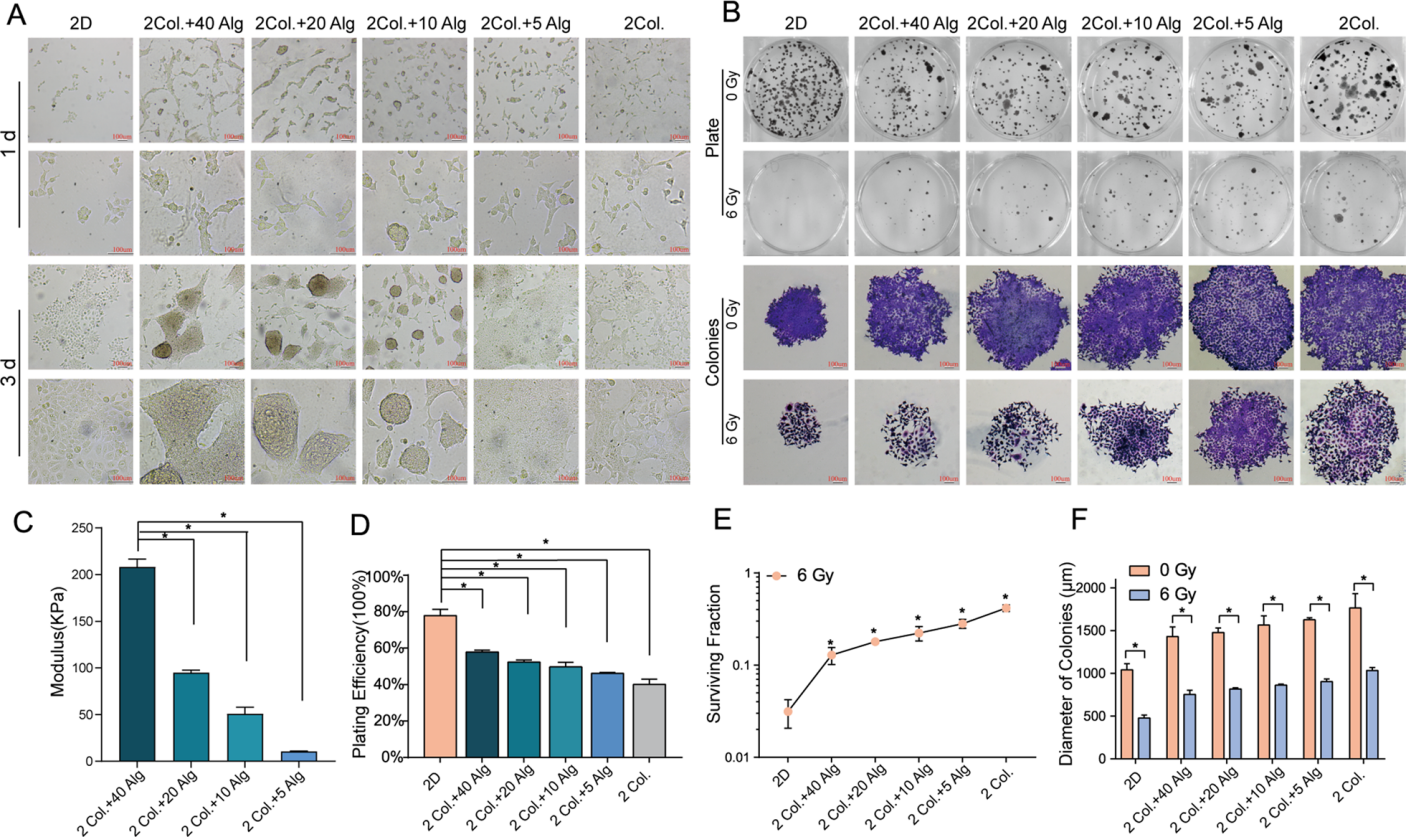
Radiation resistance decreased as the stiffness of On-Surface 3D matrix decreased A Effect of different surface stiffness of hydrogel on the morphology of CNE-1 cells on 1st, 3rd day (scale bar 100 μm). The lower concentration of sodium alginate, the more adherence, extension, connectivity of cells represented. B The formation of colonies after irradiated with 0 and 6 Gy X-rays cultured on different matrix stiffness (scale bar 100 μm). C Stiffnesses of hydrogel On-Surface 3D culture system consisting of permanent concentration of collagen and different concentrations sodium alginate. With the concentration of sodium alginate decreasing, shear stiffness declined. D The proliferation of CNE-1 cells on different stiffness substrates reflected by planting efficiency of colony formation assay. As the proportion of sodium alginate decreased (i.e., the stiffness decreased), the planting efficiency gradually descend. E The surviving fraction (6 Gy, *n* = 3, P* < 0.05) increased with the matrix stiffness declining. F Diameter of colonies increased with the matrix stiffness declining (P* < 0.05)
